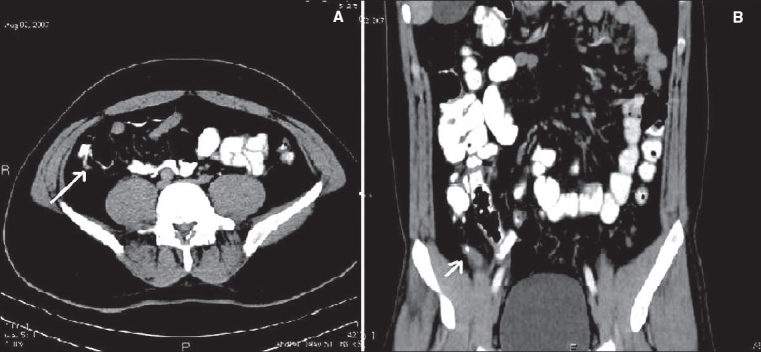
Distal appendicitis. Contrast-enhanced axial CT scan (A) shows that the proximal appendix is contrast-filled (long arrow). The coronal reformatted image (B) shows focal periappendiceal inflammatory changes and a thickened distal appendix (short arrow)
